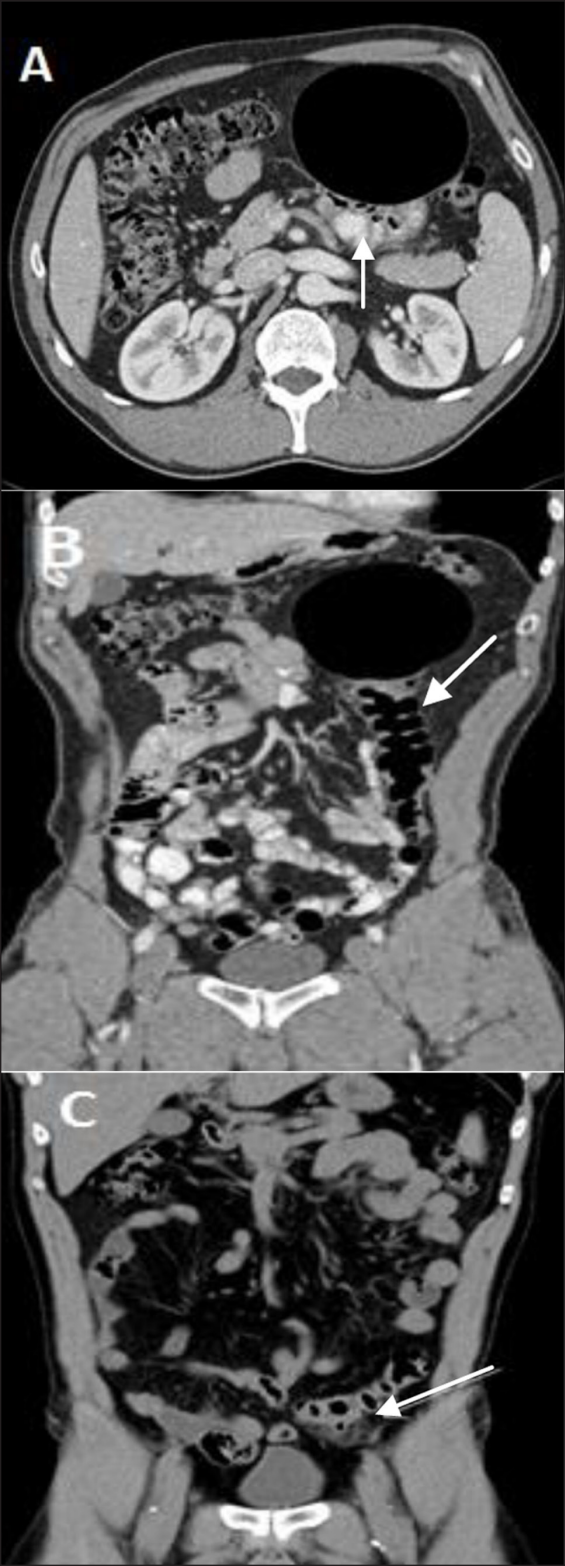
(A) and (B) Contrast-enhanced CT scan showing a 10.4 × 8.1 cm smooth-walled well-defined cystic air-filled lesion adjacent to the sigmoid (arrow) lifted in the left hypochondrium. (C) Unenhanced CT scan of the abdomen from one year earlier showed sigmoid diverticulitis (arrow) located in the left iliac fossa and without the cystic mass.
